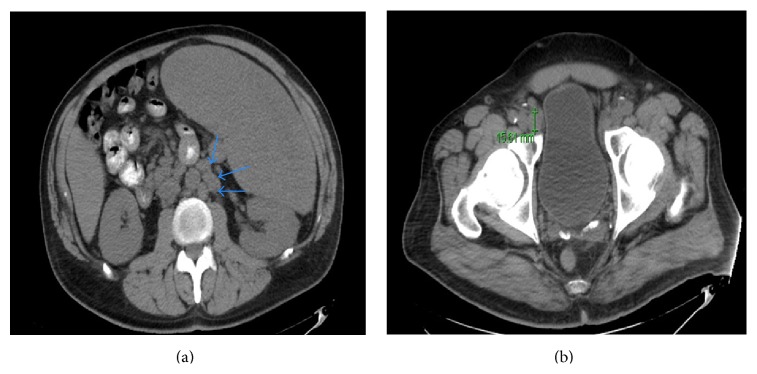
Diffuse lymphadenopathy. (a) Transverse CT of the abdomen showing enlarged para-aortic lymph nodes (blue arrows) reaching 14 mm in the shortest axis. (b) Transverse CT of the pelvis showing enlarged inguinal lymph nodes reaching 15 mm in the shortest axis.
